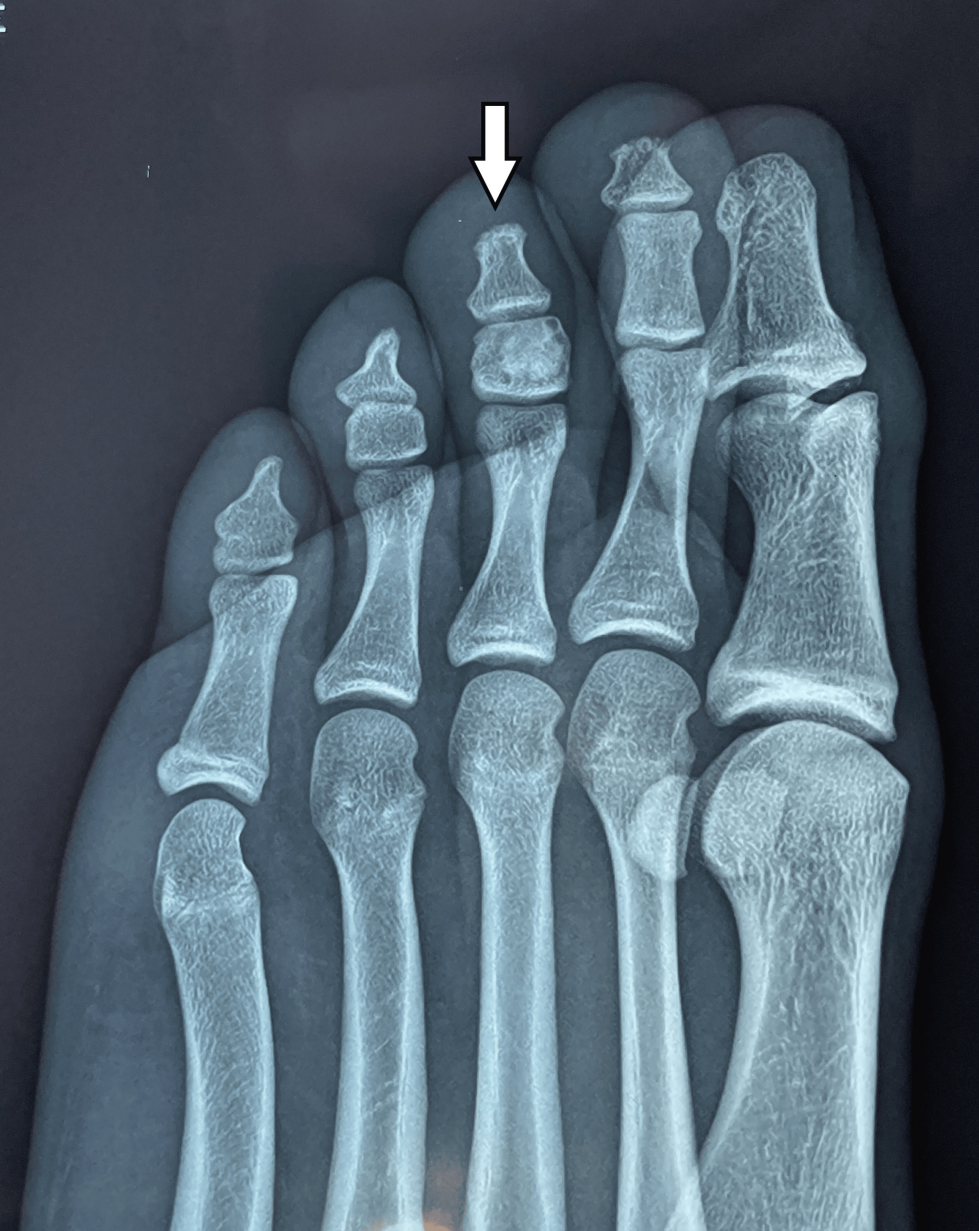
Lateral X-ray showing a central sclerotic lesion in the middle phalanx of the third toe, surrounded by a thin radiolucent zone
